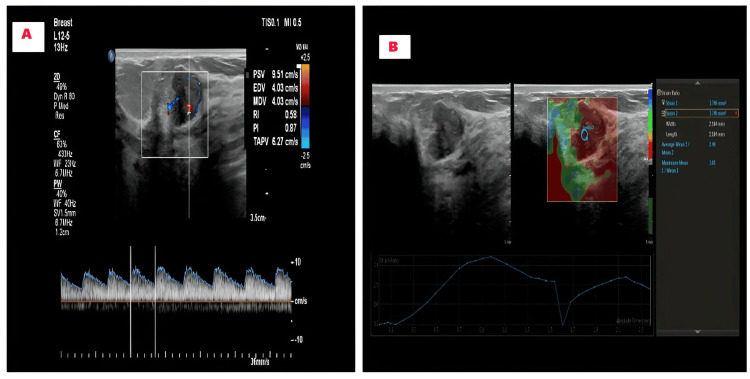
Assessing BI-RADS 3 lesions using spectral Doppler and strain elastography A: Spectral Doppler measured the PI and RI B: The strain ratio was calculated using strain elastography technique BI-RADS 3: Breast imaging reporting and data system; PI: pulsatility index; RI: resistance index.
